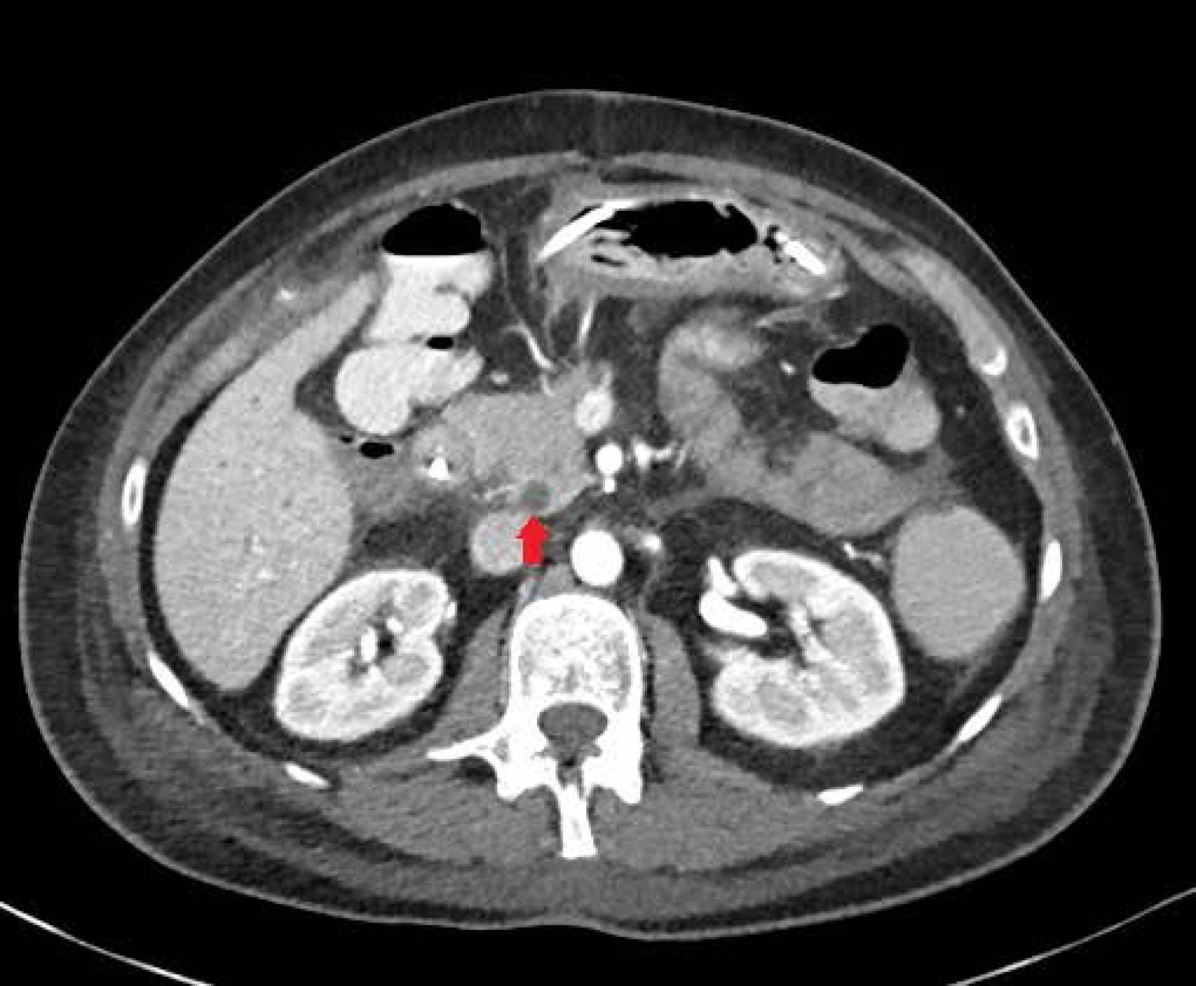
Abscess identified from CT imaging
